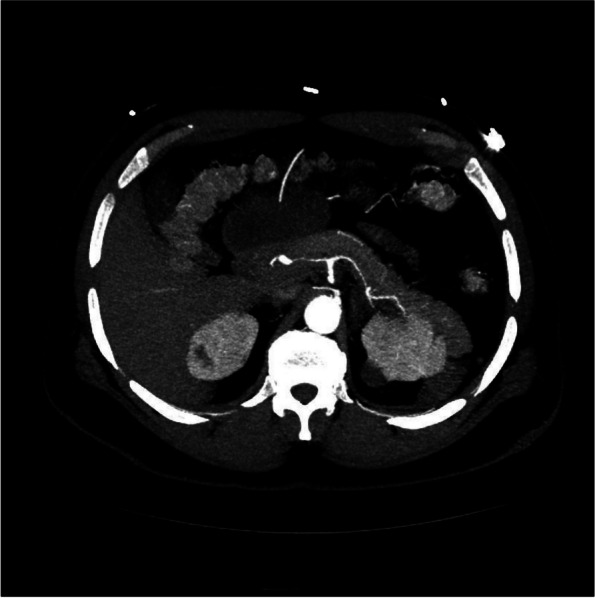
CT Angiography – Narrowing and Dilation of the celiac axis and proximal branches
